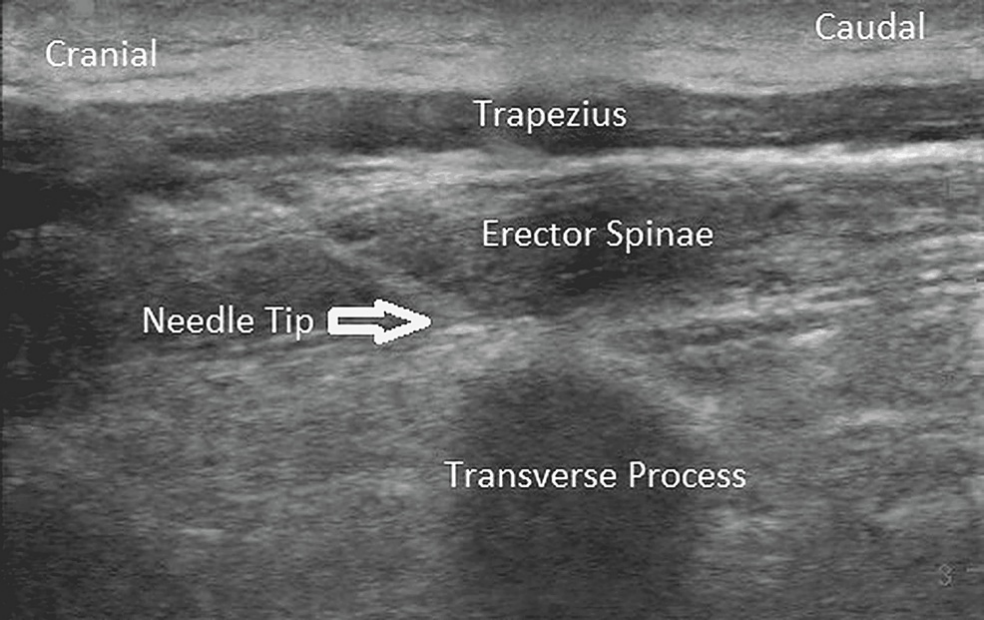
Erector spinae plane under longitudinal parasagittal ultrasound visualization; needle tip in a plane deep to the erector spinae muscle
